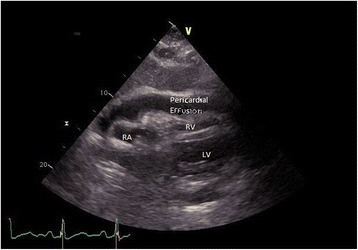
Transthoracic echocardiogram with subcostal view. Pericardial effusion with compression of the *RA* right atrium, *RV* right ventricle, *LV* left ventricle
